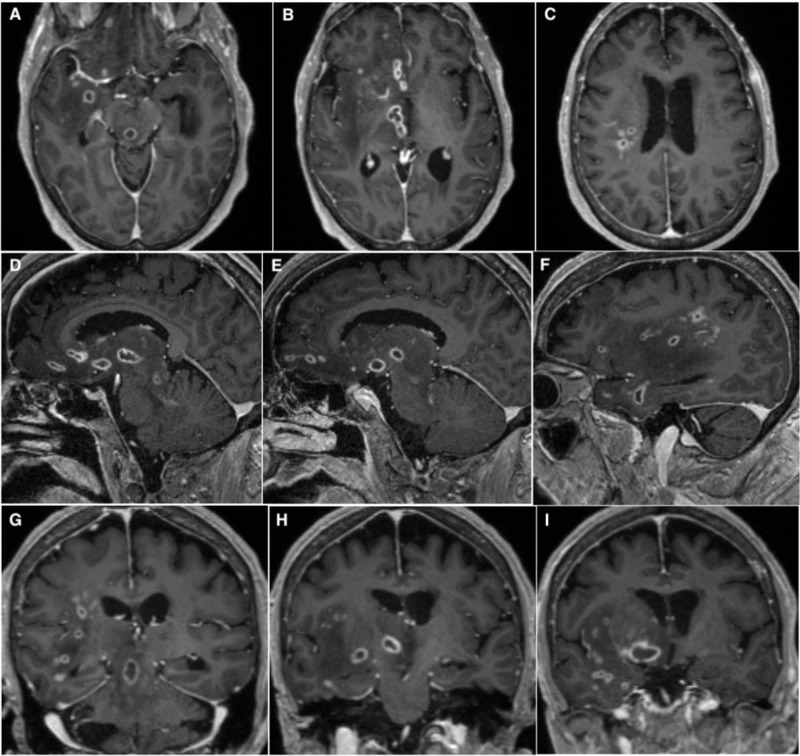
Contrast-enhanced MRI Contrast-enhanced MRI T1 sequence on axial (A-C), sagittal (D-F), and coronal (G-I) planes showing unilateral ring-enhancing lesions in the midbrain, thalamus, and frontotemporal subcortical regions.
